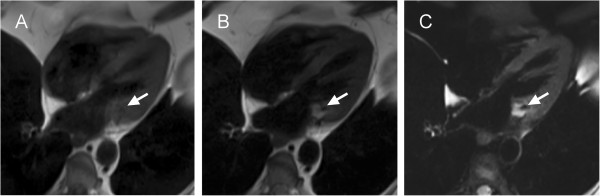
Turbo spin-echo (TSE) T1-weighted (A), TSE T2-weighted (B) and fat-saturated TSE T2-weighted (C) MR images in 4-chamber orientation. The mass is isointense to myocardium in the T1-weighted image and displays hyperintense signal on T2-weighted sequences with hypointense regions (arrows).
